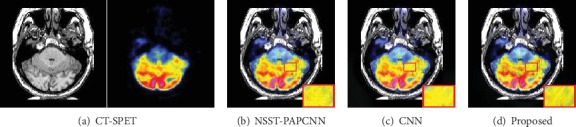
Fusion results of different methods of CT-PET image.
